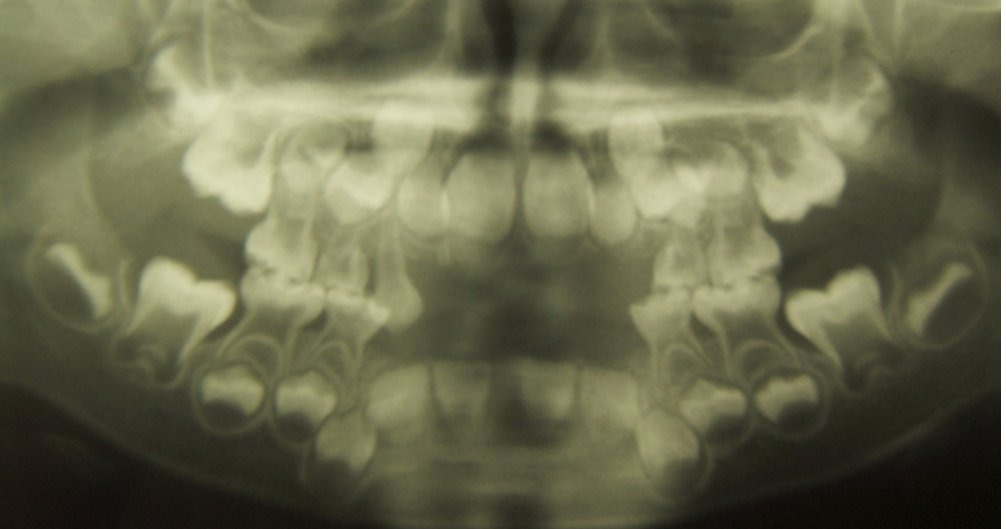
Patient 5: Panoramic radiograph at 5 years of age. The radiograph reveals enlarged pulp chambers and reduced thickness of both enamel and dentin.
